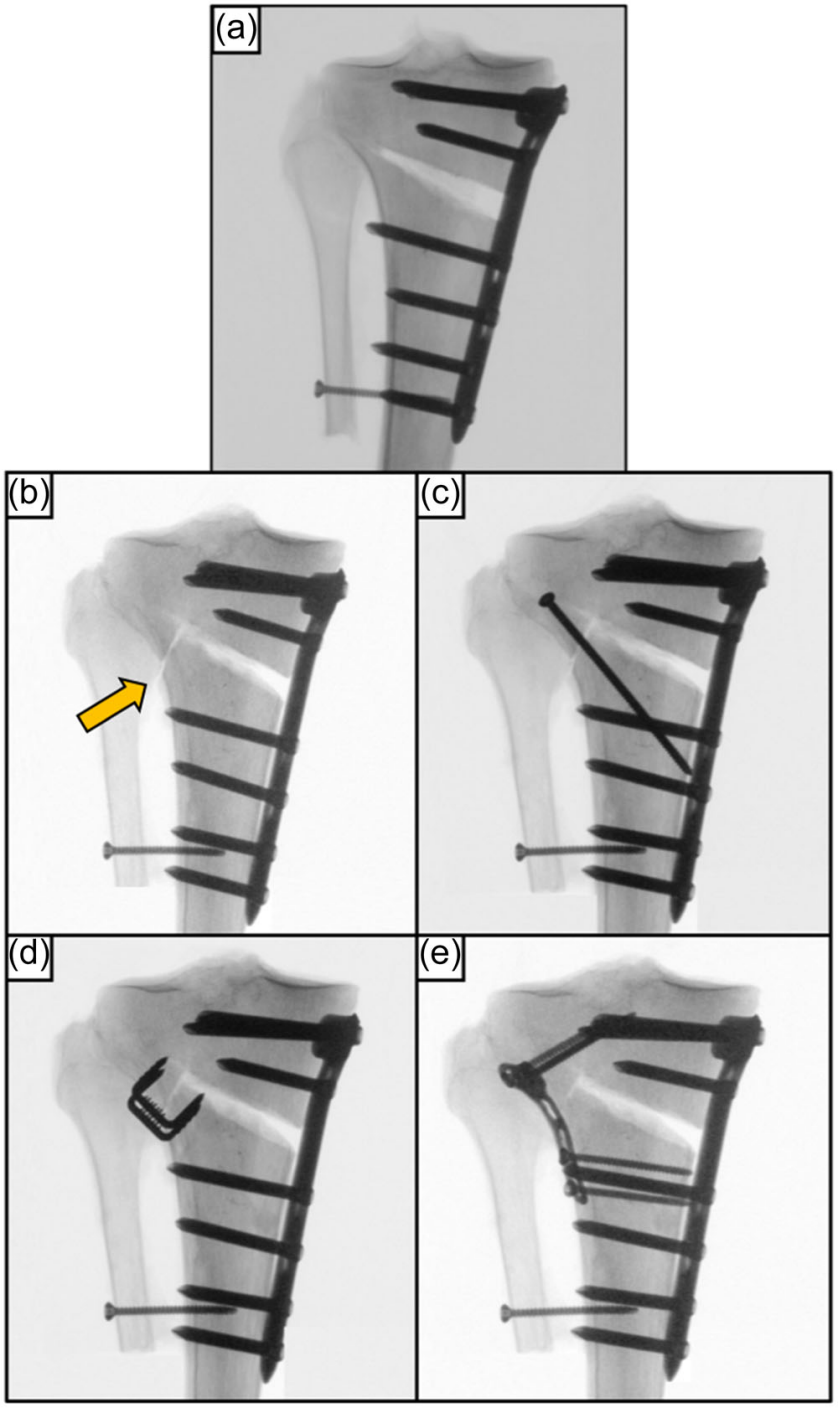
Anteroposterior radiographs of a right proximal tibia after a biplanar, medial open wedge high tibial osteotomy fixed with an ipsilateral locking compression plate system. (a) Preserved lateral hinge (intact). (b) Construct with a lateral hinge fracture (LHF) extended distal to the proximal tibiofibular joint (Takeuchi Type II fracture; orange arrow). (c) Additional lag screw fixation of the LHF (screw). (d) Additional staple fixation of the LHF (staples). (e) Additional T‐plate fixation of the LHF (plate).
